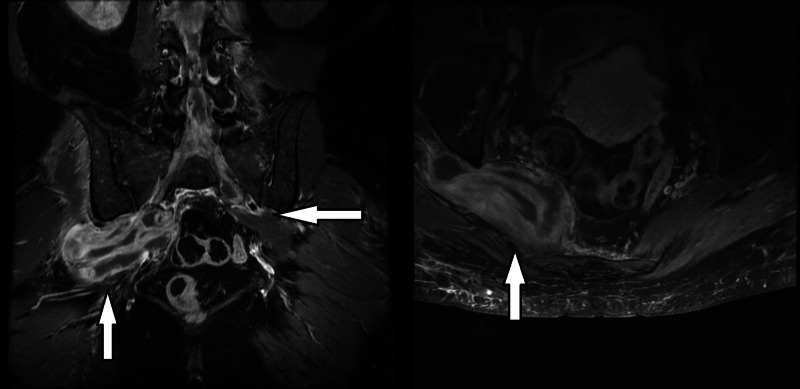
Sagittal and axial T1-weighted MRI obtained preoperatively showing a large intrapelvic abscess on the right side and a small abscess on the left side. MRI: magnetic resonance imaging
